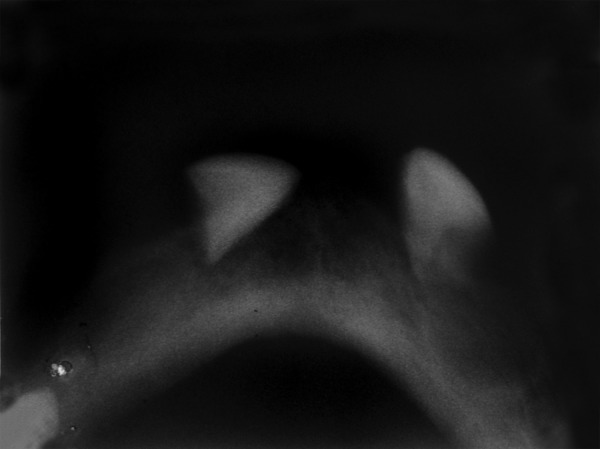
Mandibular occlusal radiographs showing multiple missing teeth along with the permanent tooth buds
